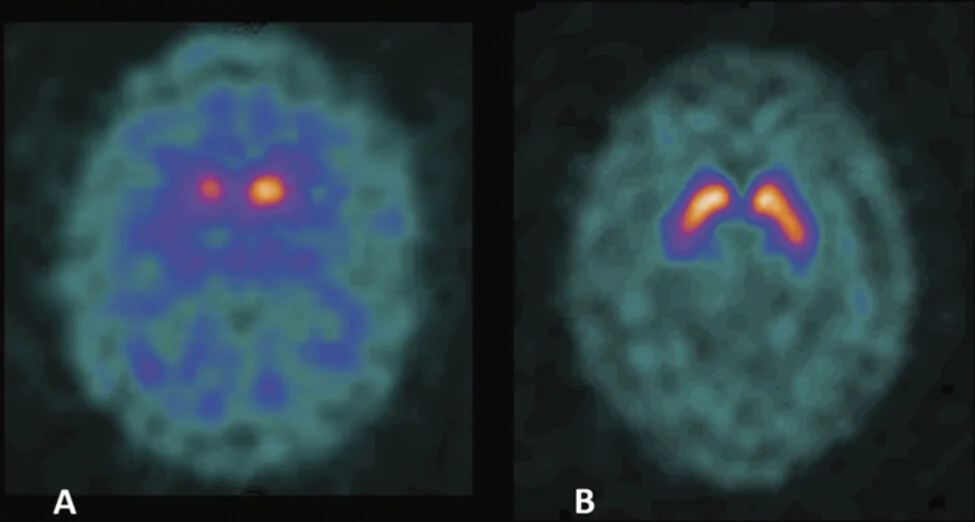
Abnormal and normal [123I]FP-CIT-SPECT scans. (
